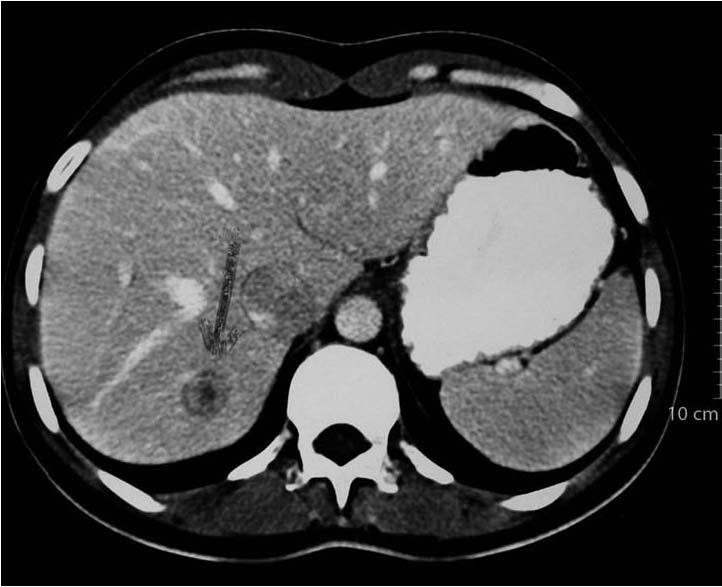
Computed tomography scan of metastatic lesion in the liver taken in 2002.
